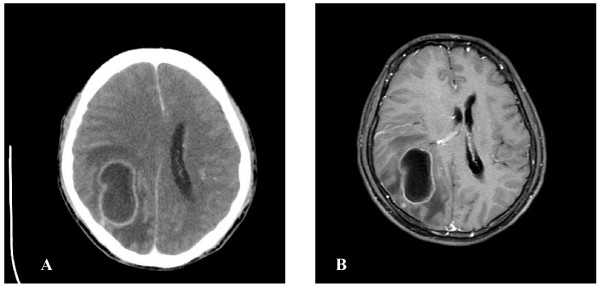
A. Axial brain CT after contrast medium injection showing a ring-enhancement lesion with perifocal edema over right occipital area. B. Axial T1-weighted MR image of the brain after gadolinium injection revealed ring-enhancing cystic lesion with mass effect suggestive of right occipital abscess
